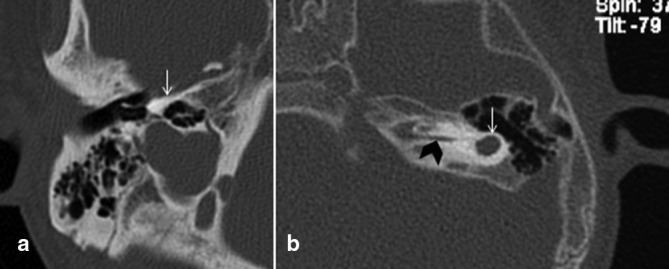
Axial CT showing complete labyrinthine aplasia in patient with LAMM syndrome (Congenital deafness with labyrinthine aplasia, microtia, and microdontia), no labyrinthine is seen in a small and dense otic capsule (arrow). Figure 4B Rudimentary otocyst (white arrow) with narrowed IAC (black arrowhead). IAC, internal auditory canal.
